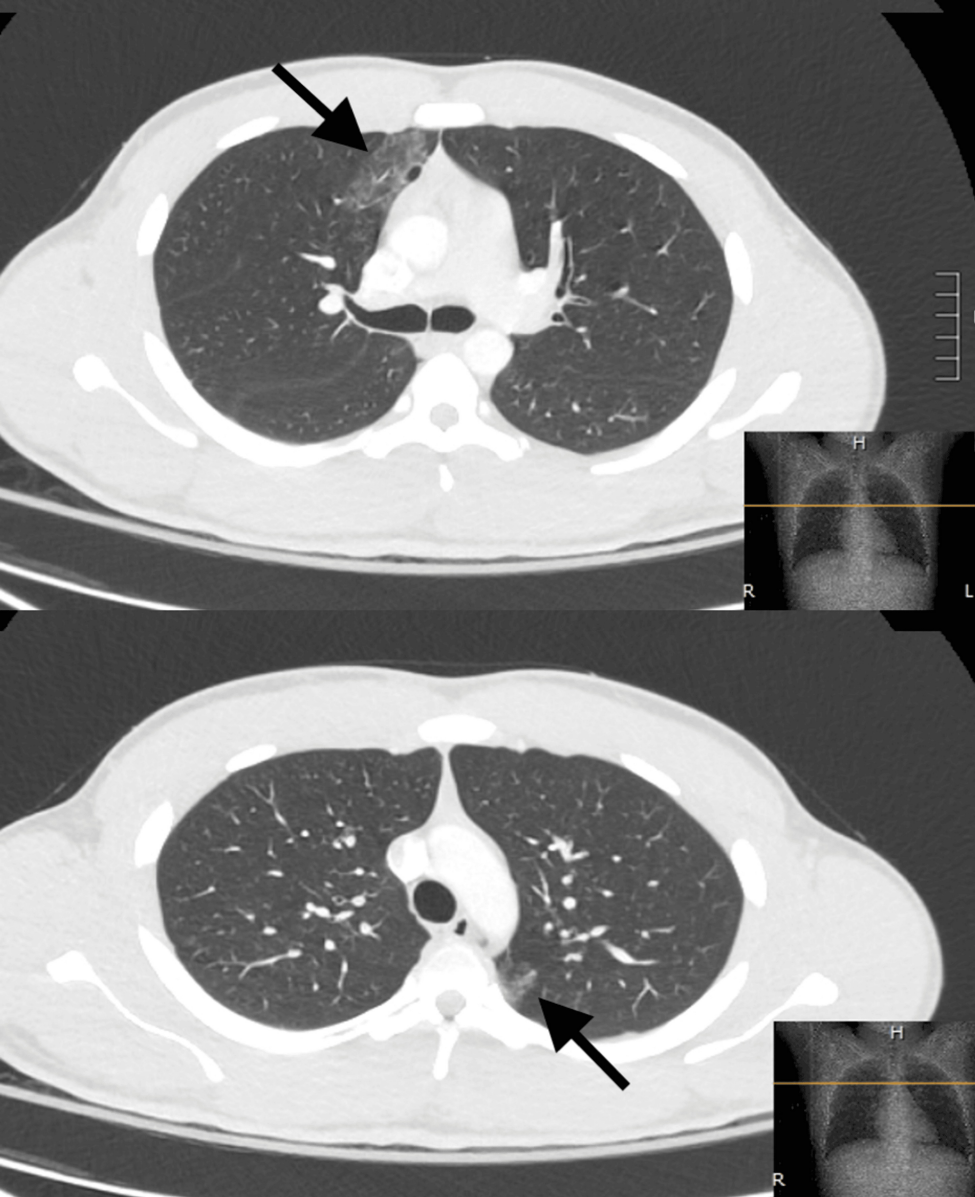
Computed tomography (CT) scan of the chest Axial images seen in lung windows from computed tomography (CT) scan of the chest with intravenous contrast enhancement revealing small ground glass opacities suggestive of pulmonary contusions (arrows).
